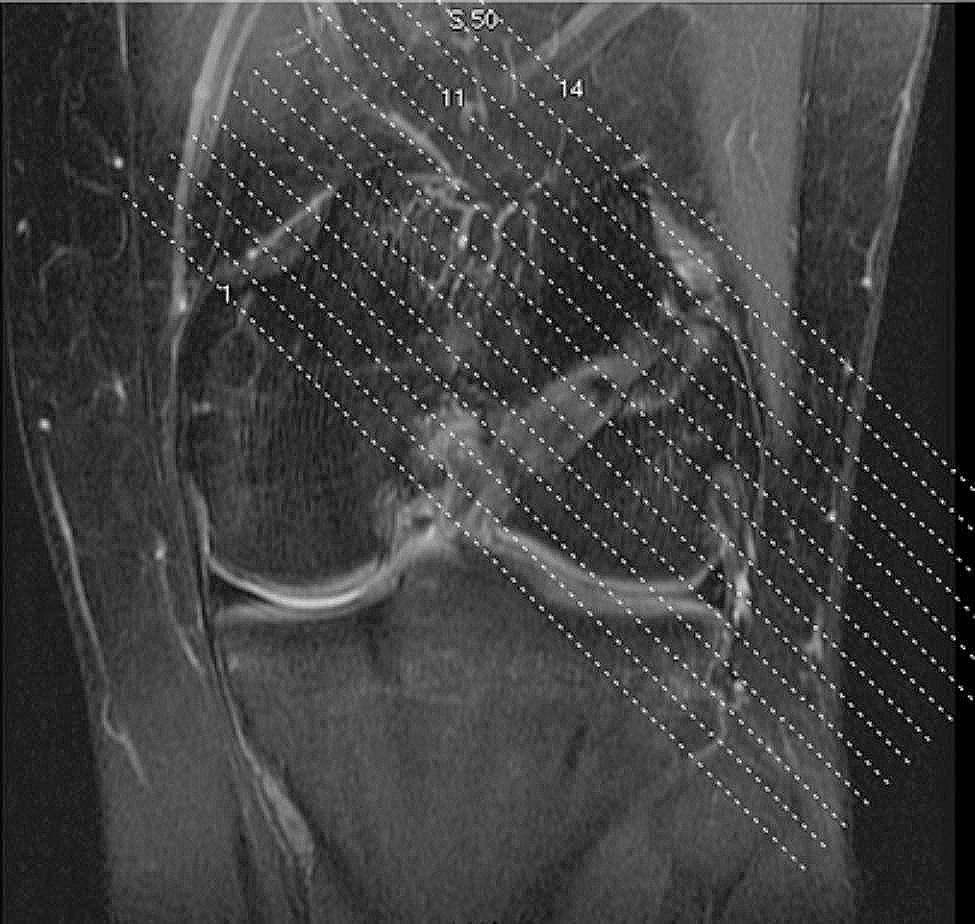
MRI of the knee 6 months after ACL reconstruction: This is a localized scan image perpendicular to the femoral bone tunnel of the knee joint(MRI has a scanning layer thickness of 5 mm)
